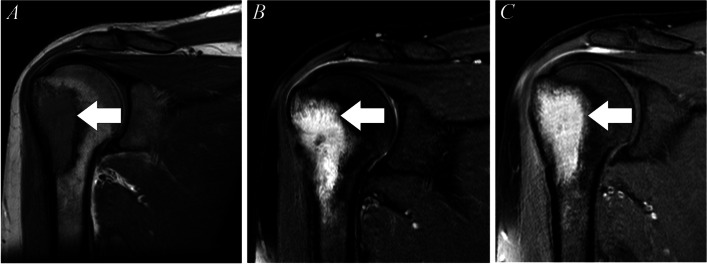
MRI of right shoulder (1) showing ill-defined area of signal abnormality involving the lateral aspect of the greater tuberosity (arrow). It demonstrates a low signal intensity on T1 (A) and a bright signal on the T2 fat-saturated images (B). On the post-contrast image (C), the majority of the intramedullary component demonstrates thick peripheral rim enhancement and areas of solid enhancement, indicating osteomyelitis
